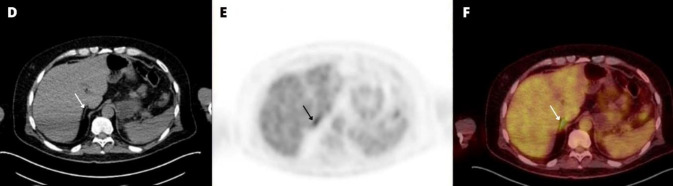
Axial CT (D) showed a normal-sized right adrenal gland (arrow), while the PET (E) and fused (F) showed prominent right adrenal gland FDG uptake (arrows, SUV_max_=5.6) that was almost two times higher than the physiologic liver FDG activity
